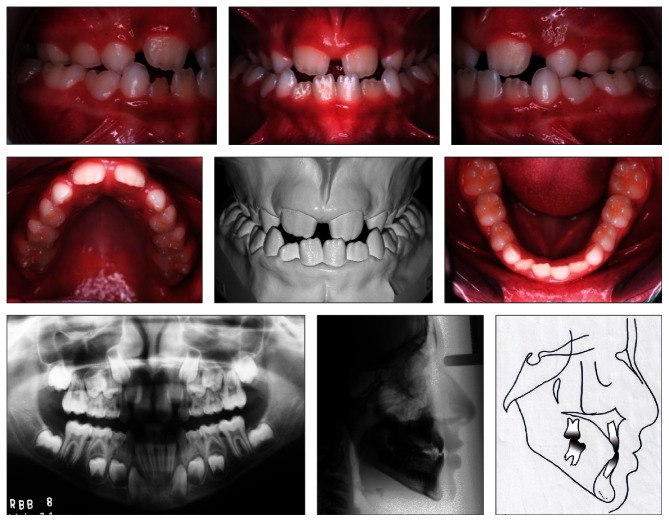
Pretreatment intraoral photographs, panoramic and cephalometric radiographs, and cephalometric tracing. Angle Class III malocclusion. Complete maxillary crossbite.
